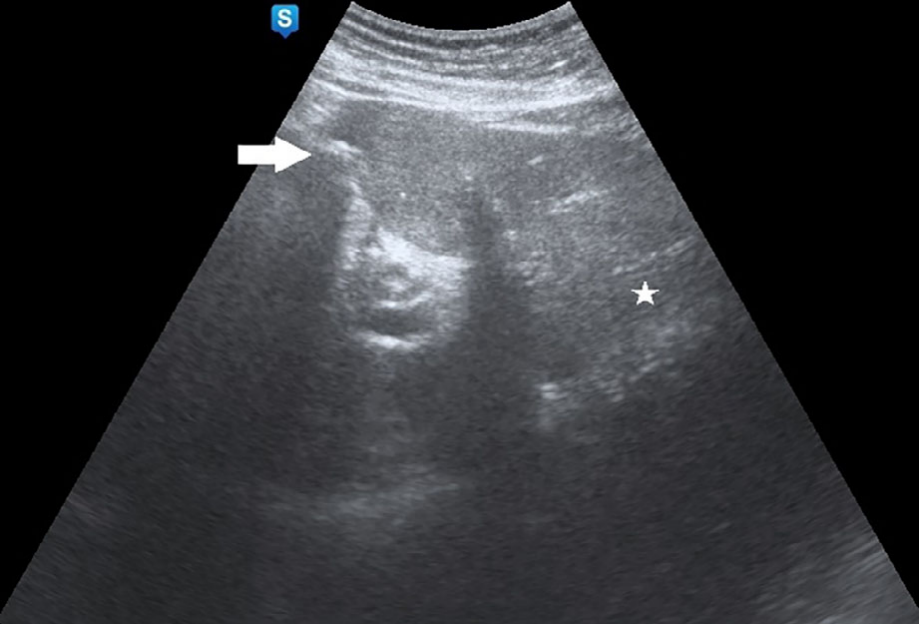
Transverse sonogram of the expected location of the duodenal bulb shows a curvilinear focus of increased echogenicity with posterior shadowing (arrow), findings consistent with a large gallstone with multiple foci of hyperechogenicity in the left liver lobe with ring‐down artifact (star), which suggests pneumobilia.
